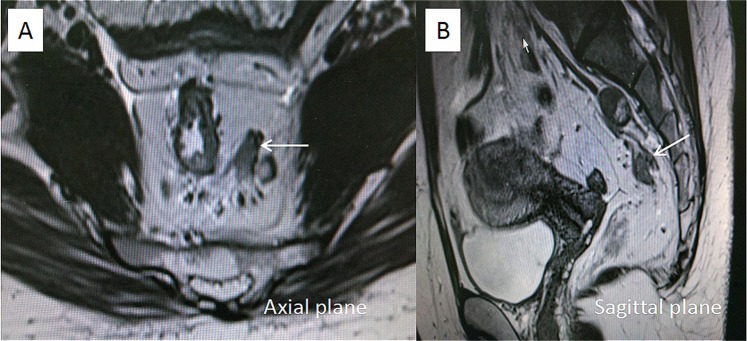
T2-weighted axial (A) and sagittal (B) magnetic resonance images. Magnetic resonance imaging-detected EMVI (MR-EMVI) score = 4: an obvious irregular vessel with definite tumor signal (the white arrow).
